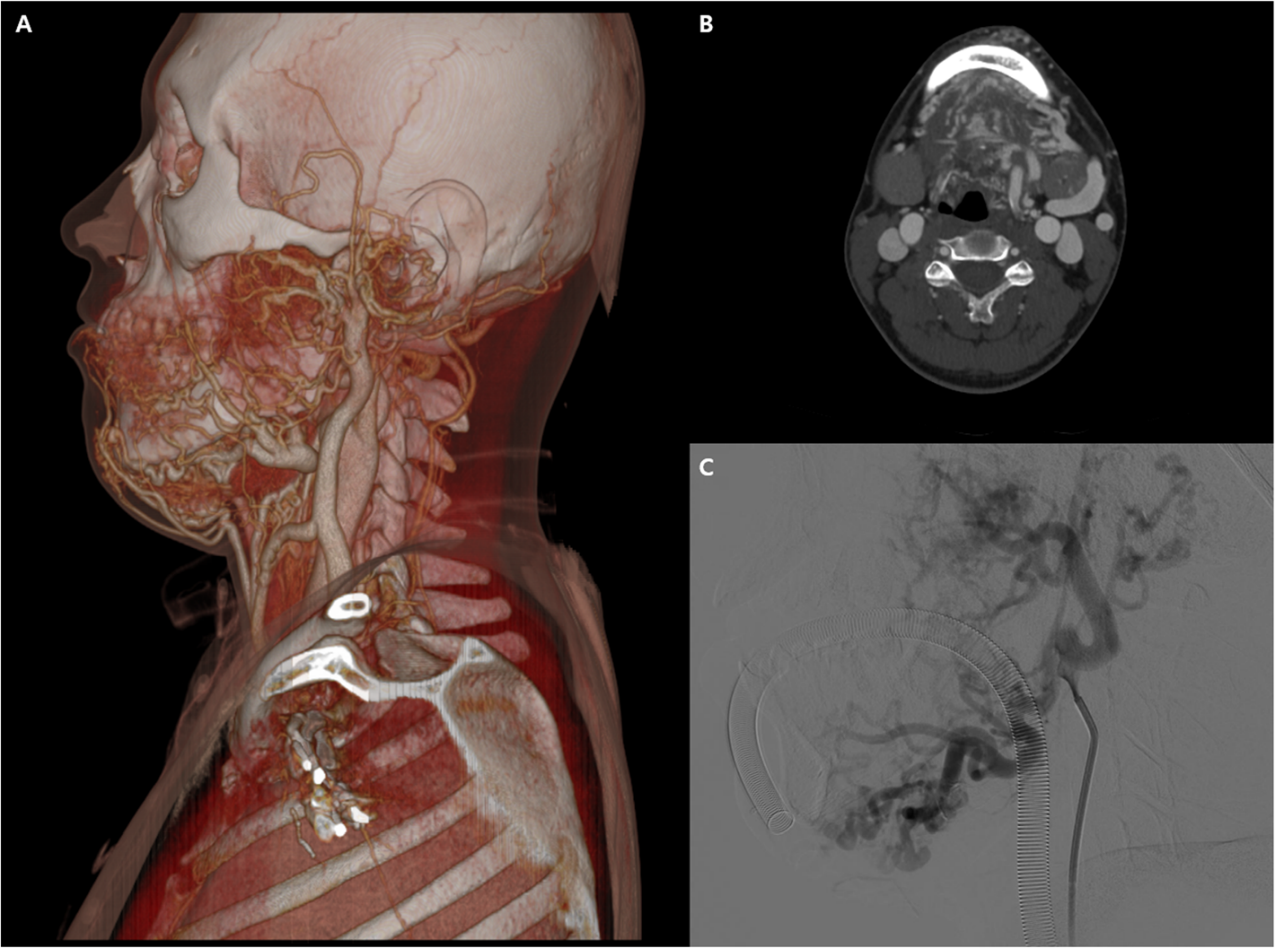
Volume-rendered image (A) and axial image (B) of CT angiography show huge AVM involving the left side of his face, jaw, tongue, neck, and left ear. C Initially performed external carotid artery angiography reveals a very large AVM, which has multiple shunts between the arterioles and venules with dilated fistulae
